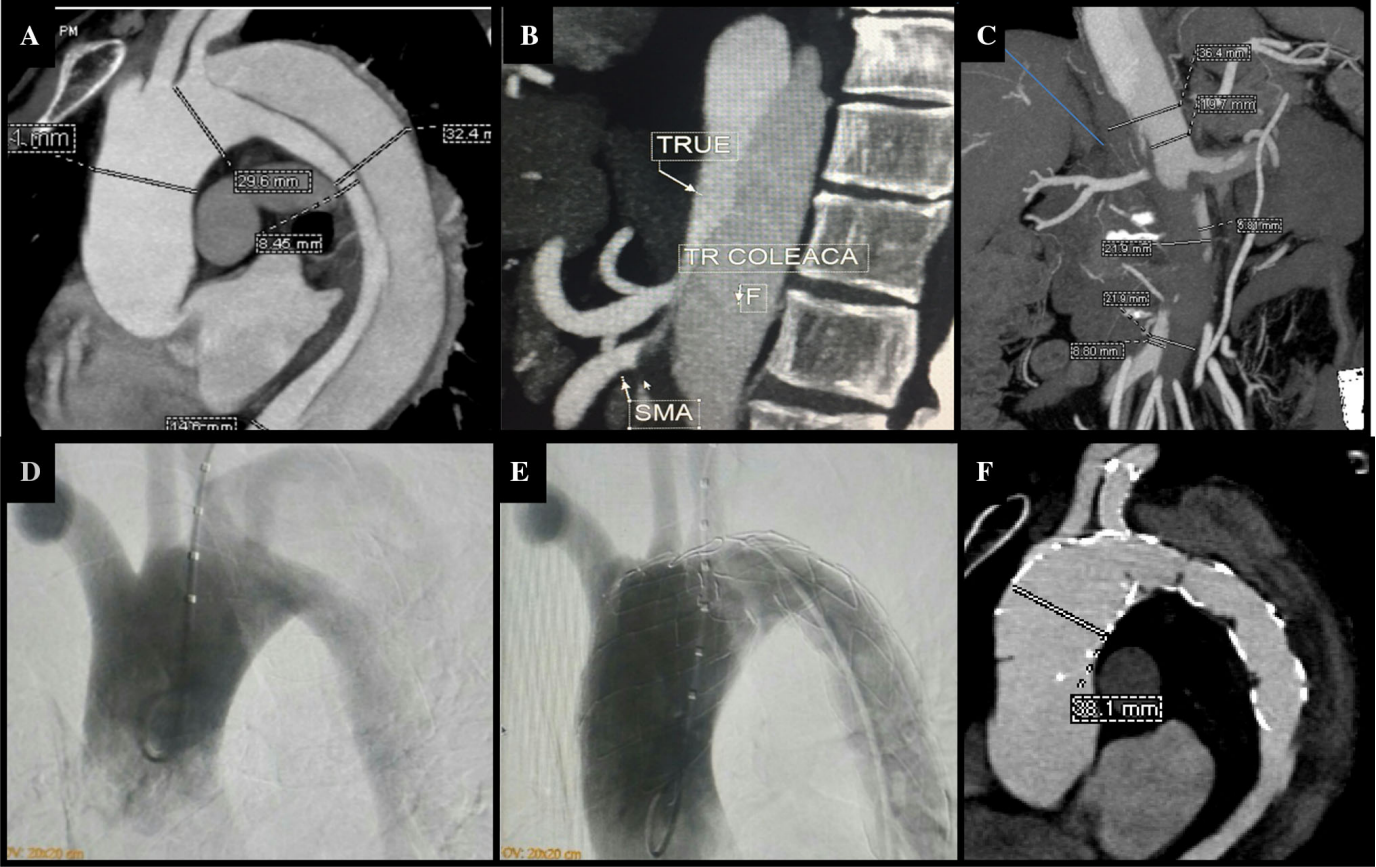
Aortic CT of the second patient showing type B aortic dissection (A) with mal-perfusion syndrome due to partial/total occlusion of the celiac trunk, superior mesenteric artery (B), renal artery and distal abdominal aorta (C). Aortogram before (D) and after the fenestrated TEVAR (E). The entry tear was located very close to the LSA. After implantation of the fenestrated stent graft, the LSA stent was patent, the entry tear was closed and the false lumen was excluded. 6 months follow up CT showed patent LSA stent and partial thrombosis of the false lumen (F)
